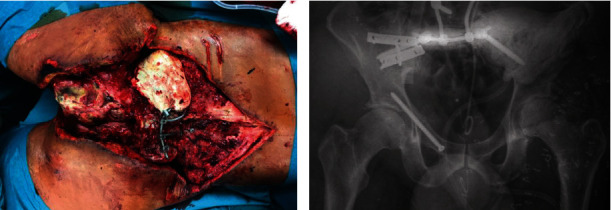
(a) Intraoperative situation after partial resection of the left iliac bone. (b) Anteroposterior X-ray of the pelvis with the bone cement spacer in place.
